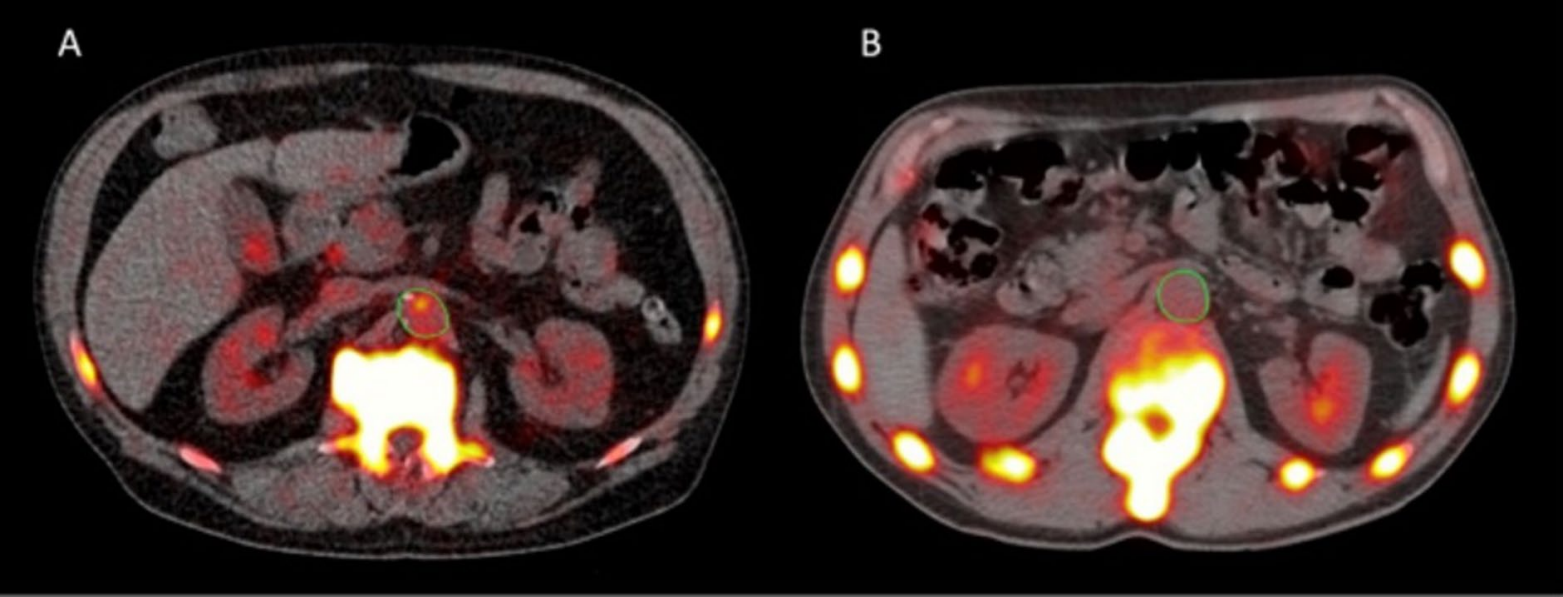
In this [^18^F]-NaF PET/CT study, regions of interest were manually outlined around the abdominal aorta, as shown in green. Comparison was made between the abdominal aortic wall of a 63-year-old patient with rheumatoid arthritis and an age- and sex-matched healthy control. The [^18^F]-NaF PET/CT scan revealed higher levels of [^18^F]-NaF activity in the abdominal aorta of the RA patient when compared to the healthy control. With permission from reference [20]
